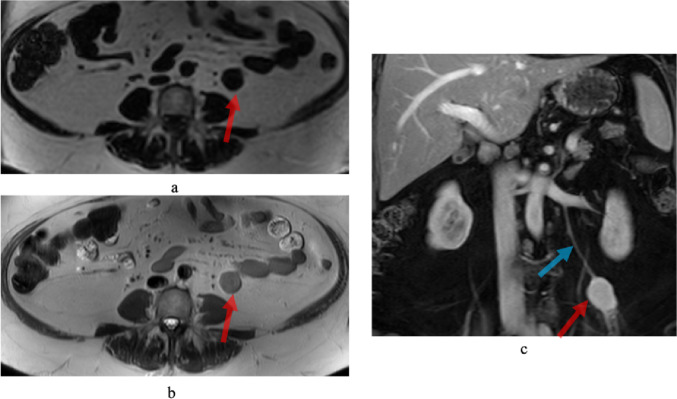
Axial T1 and T2-weighted images (a, b) without fat saturation demonstrate the mass to be T1 hypointense and moderately T2 hyperintense (red arrows). A coronal fat-saturated T1-weighted contrast enhanced image (c) demonstrates the mass (red arrow) to be avidly enhancing and contiguous with the left ovarian vein (blue arrow)
